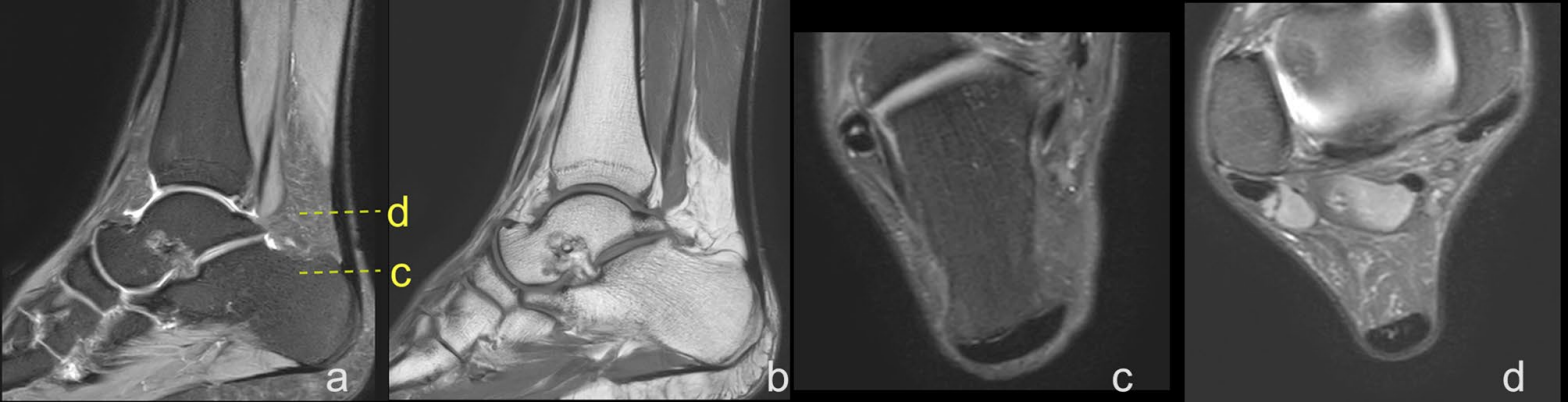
The normal Achilles tendon. A 34-year-old female with clinical suspicion of a nerve tumor on n. tibialis. (a) PD- weighted SPAIR, (b) T1-weighted, (c) and (d) PD- weighted TSE SPAIR.
